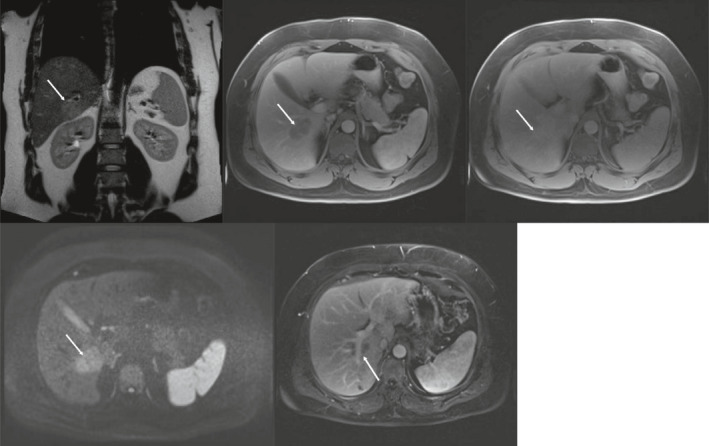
MRI findings upon initial presentation. MRI of the liver shows a large nodule in segment 5‐6 (right to left, top row). The nodule is T2 hyperintense, hypointense on T1‐weighed, contrast‐enhanced, portovenous phase images and shows enhancement in the delayed phase (right to left, bottom row). The nodule exhibits diffusion restriction and a branch of the portal vein passes through the lesion
